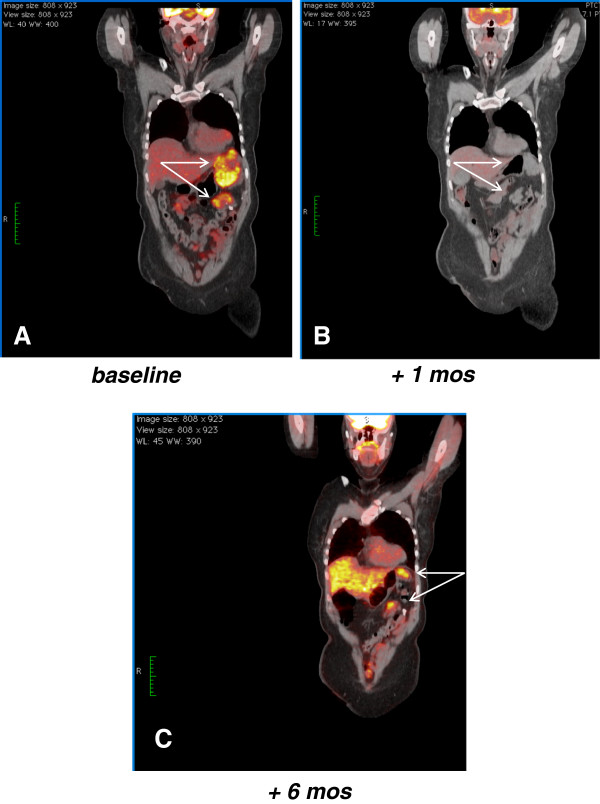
[18F]fluorodeoxyglucose–positron emission tomography scan (PET) tumor assessment. In panels A and B (white arrows) PET shows a marked decrease in maximum SUV comparing the tumor before and after 4 weeks of treatment. Panel C shows an interval tumor progression at 6 months from baseline, i.e. after 2 months from sunitinib interruption and treatment with antibiotics.
